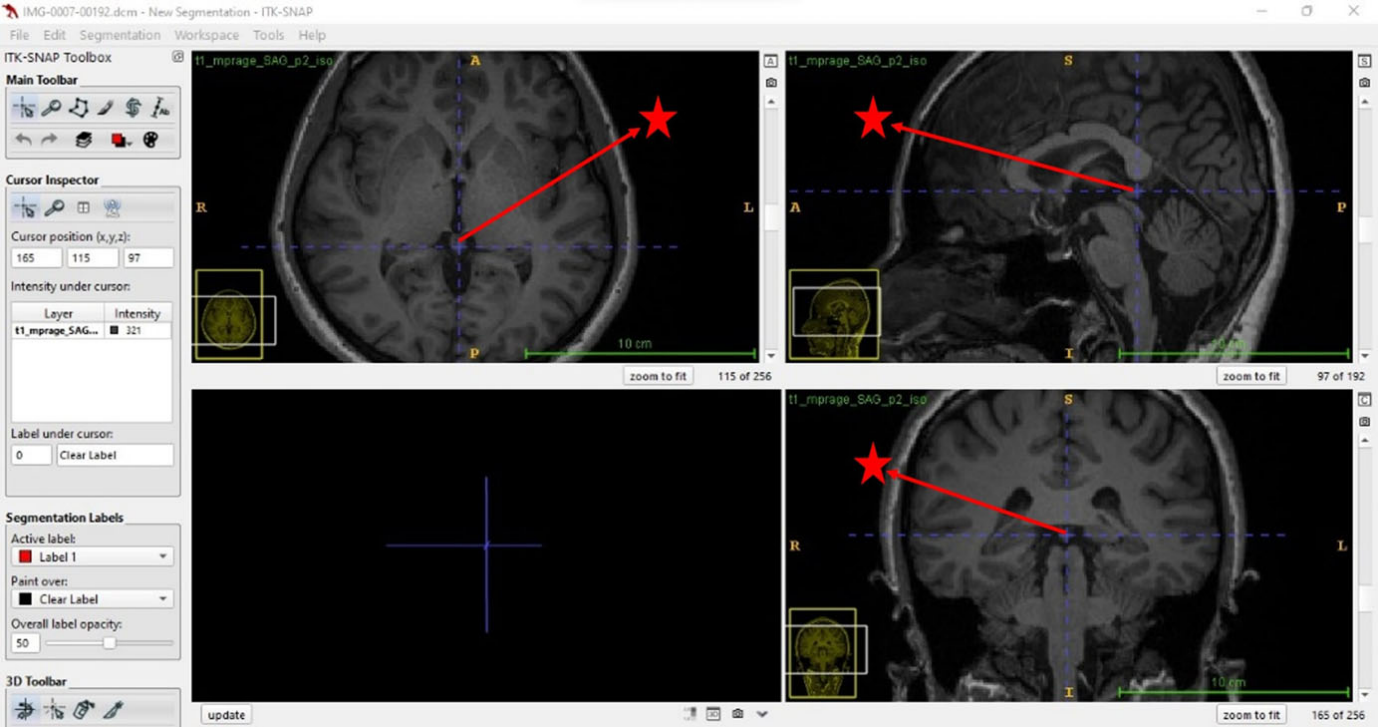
Identification of the pineal gland according to anatomical landmarks in T1-weighted magnetisation prepared with magnetisation-prepared gradient echo with rapid acquisition sequence (Red star: Pineal Gland).
